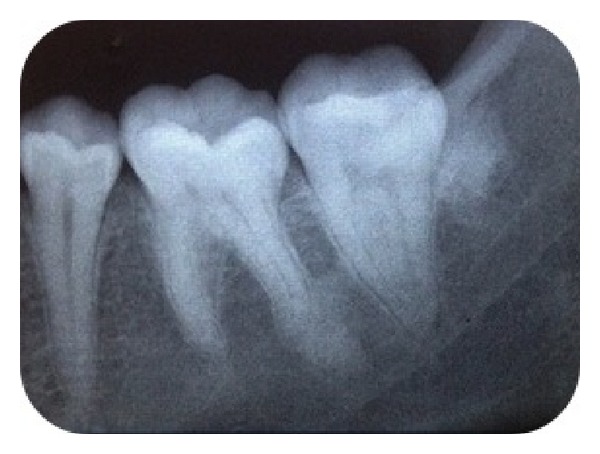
Periapical radiograph of the left mandibular molar region, showing the osteosclerotic area.
